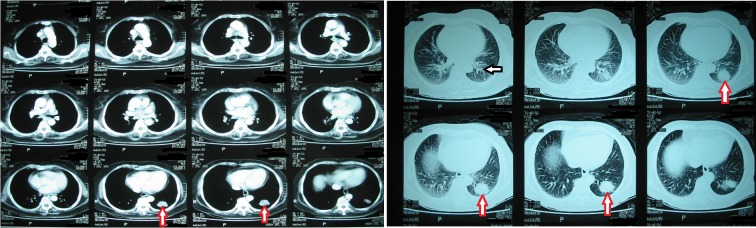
CT scan of chest A CT scan of the chest shows a 3.1 x 2.9 cm mass (red arrow) in the left lower lobe and mediastinal lymphadenopathy (black arrow).
